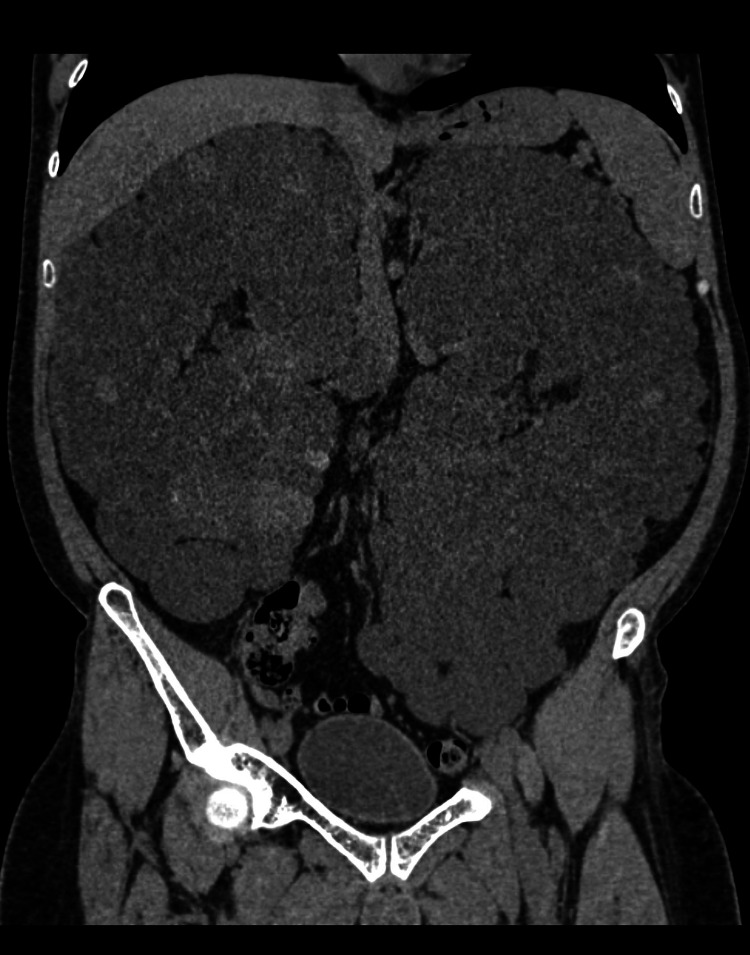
Abdominal CT scan showing markedly enlarged kidneys bilaterally extending downwards to the pelvis with innumerable multiple variable-sized renal cysts. Right kidney measures about 29cm, and the left kidney measures about 33cm.
